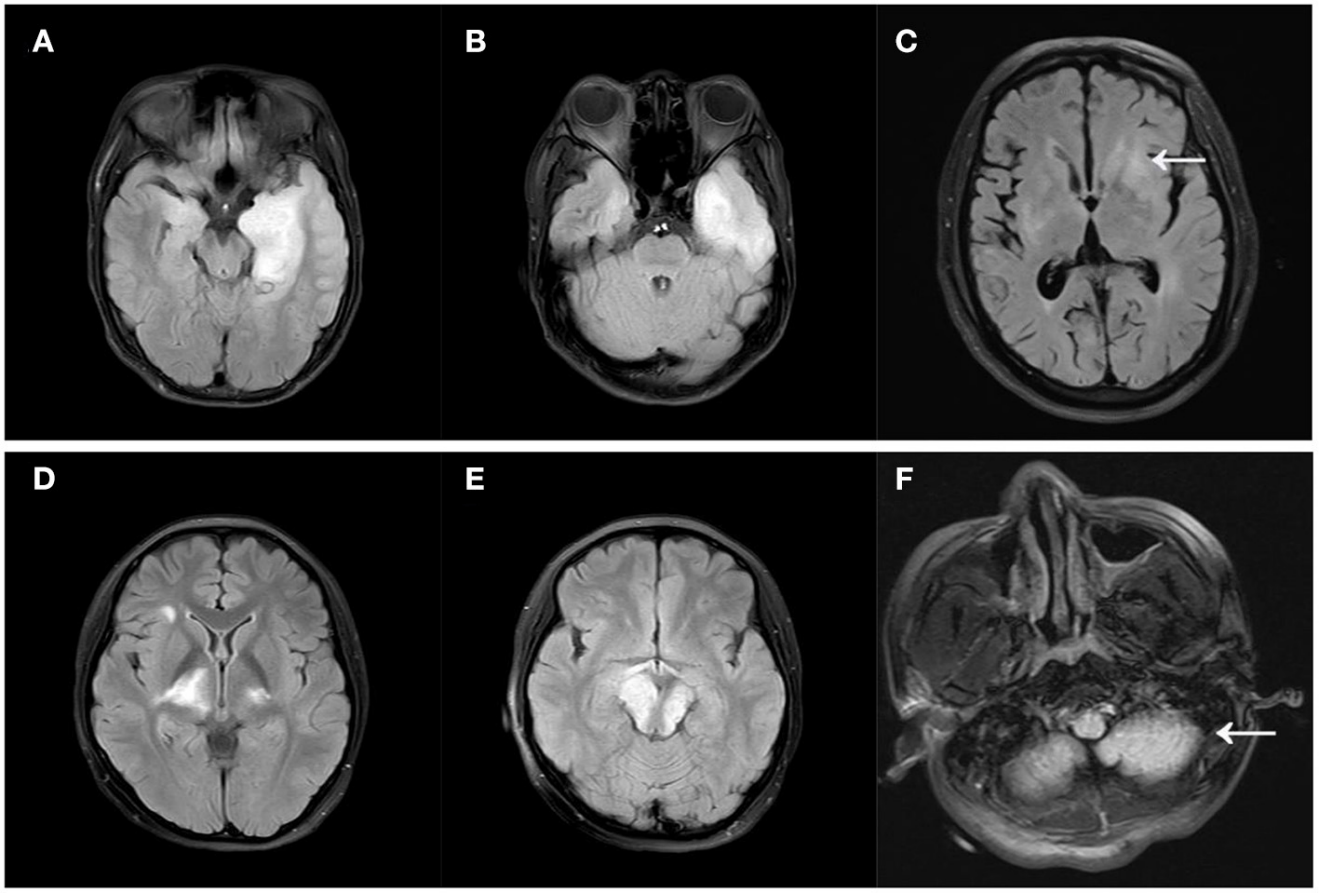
Different MRI types of autoimmune encephalitis. (A) Limbic encephalitis; (B) cortical/subcortical encephalitis; (C) striatal encephalitis (arrow); (D) diencephalic encephalitis; (E) brainstem encephalitis; (F) cerebellar encephalitis (arrow). All MRIs were FLAIR images.
